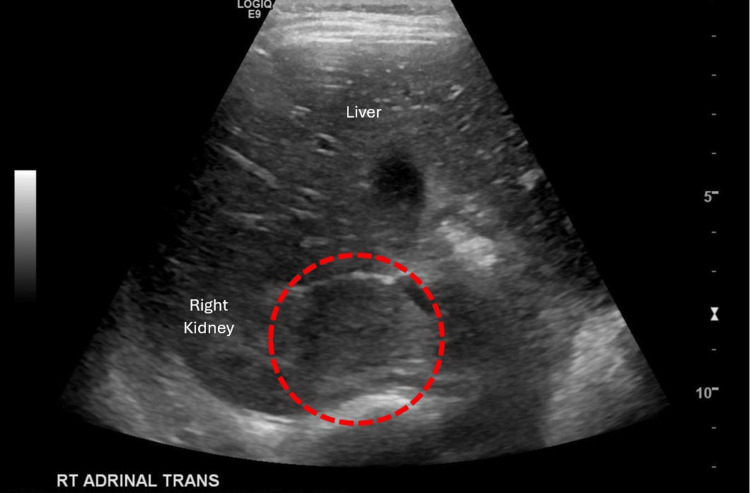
Abdominal ultrasound still image demonstrating 5.3 x 3.3 x 4.0 cm right suprarenal mass (red circle).
